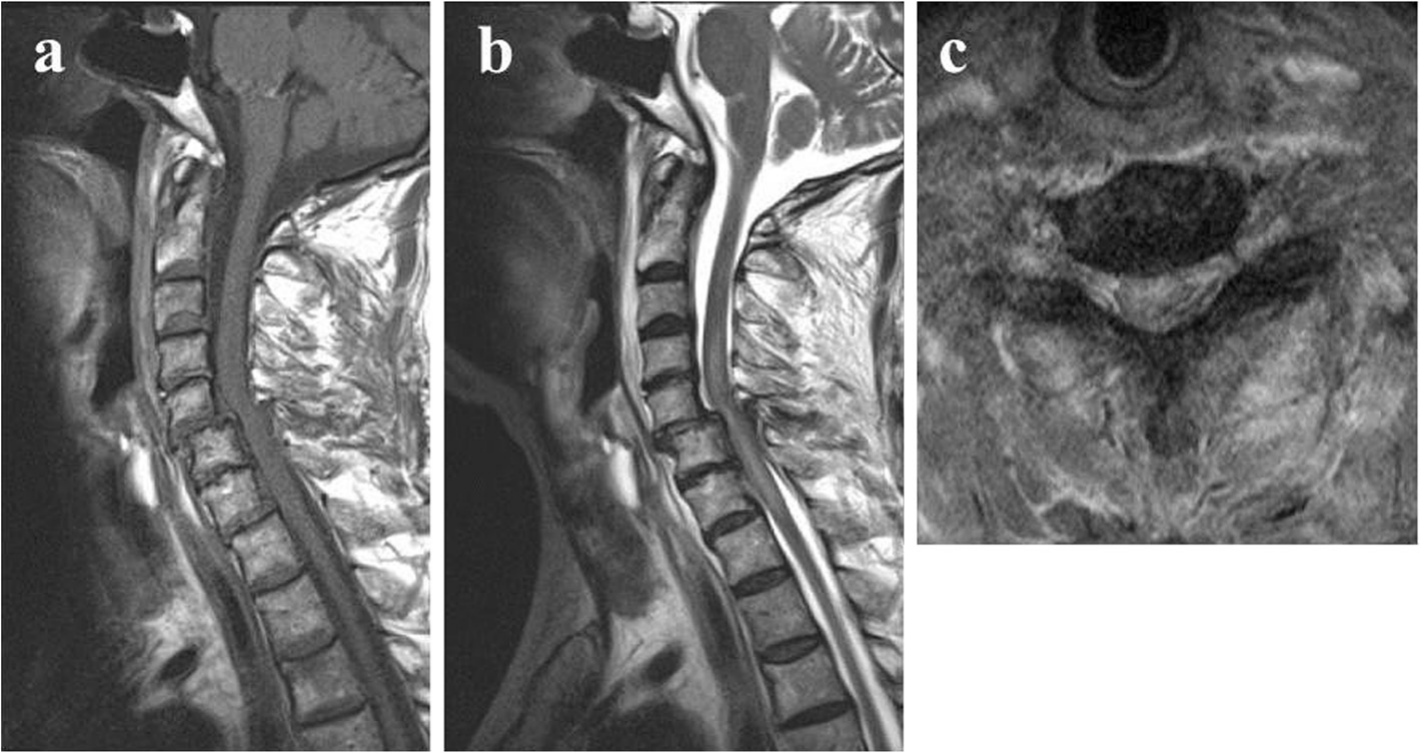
Magnetic resonance imaging showing spinal canal stenosis and swelling of the spinal cord eight weeks after the traffic accident. (a) T1-weighted sagittal image; (b) T2-weighted sagittal image; (c) T2-weighted axial image.
